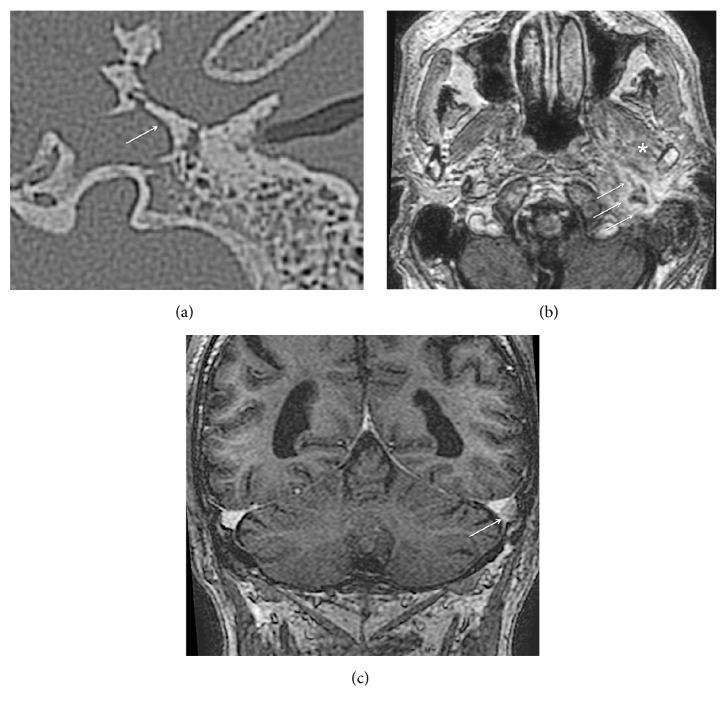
(a) CT scan of the left temporal bone—a bony sequestrum in the tympanic part is shown by the arrow. (b) An abscess in the left infratemporal fossa appears as a contrast-enhanced mass on the periphery, with irregular contours (arrows). A trismus was explained by inflammatory infiltration of the lateral pterygoid muscles (asterisk). (c) A thrombosed sigmoid sinus is seen on the left side (arrow).
